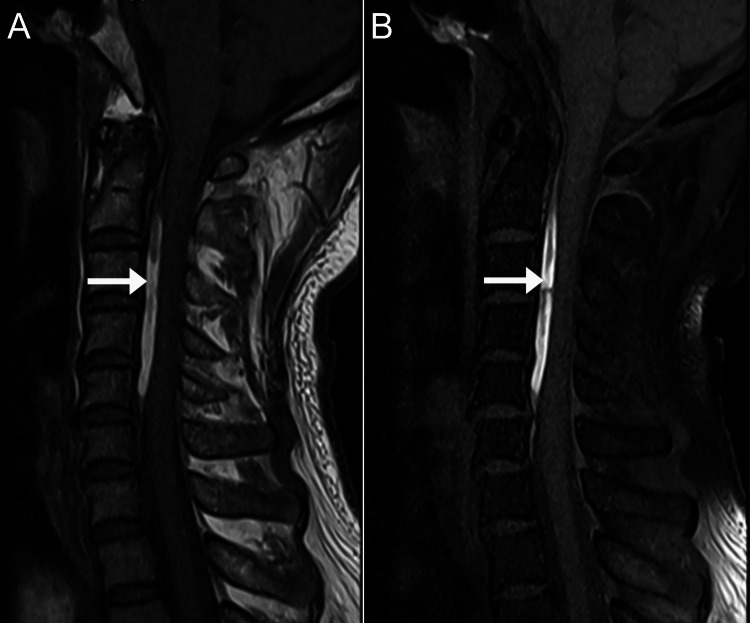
Magnetic resonance imaging of the cervical spine, mid-sagittal T1-weighted images A: Shows a linear hyperintense layer in the anterior epidural space spanning the vertebral levels C2 to C5 measuring 1.2 x 0.4 x 5.4 cm (arrow). B: On a fat-suppressed sequence, this layer remains hyperintense (arrow), which suggests there is no macroscopic epidural fat within this layer.
